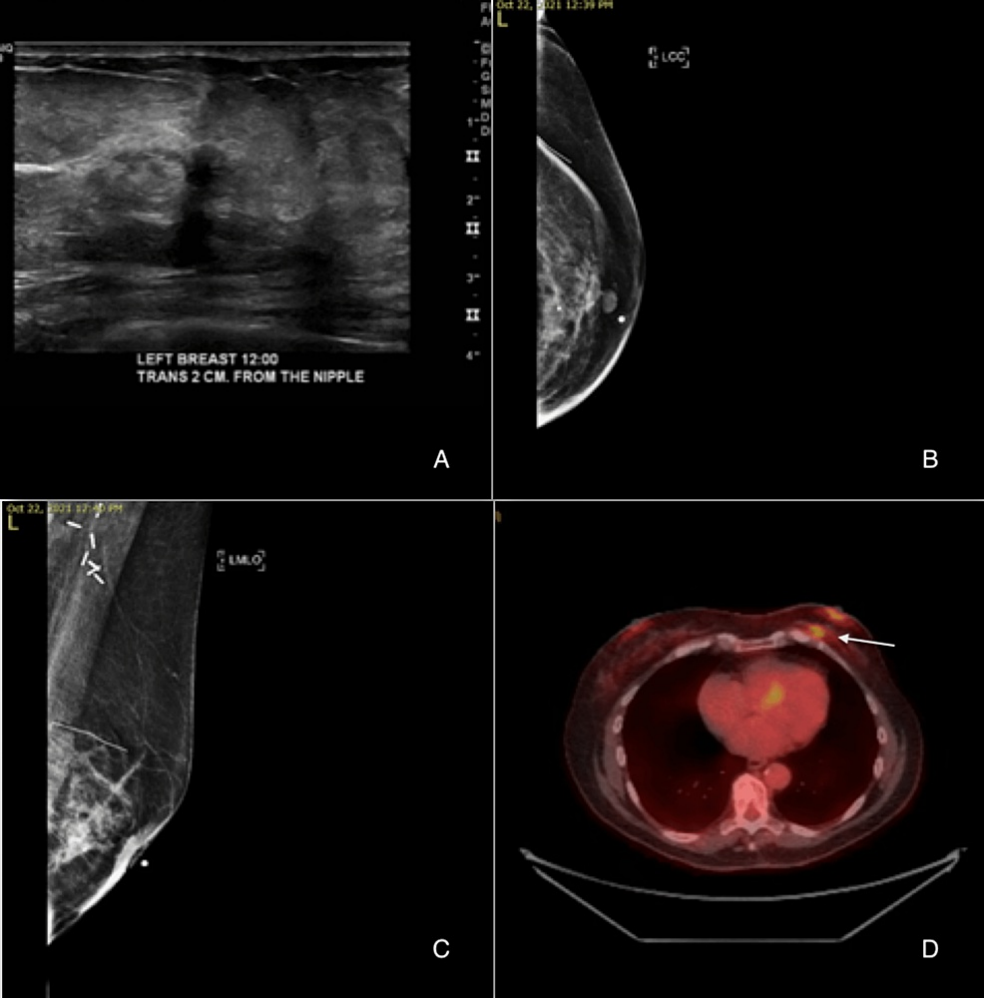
Imaging results. (A) Negative breast ultrasound. (B) Negative left breast mammogram in the mediolateral oblique view. (C) Negative left breast mammogram in the craniocaudal view. (D) PET scan findings displaying a 2-cm lesion in the left breast (arrow).
